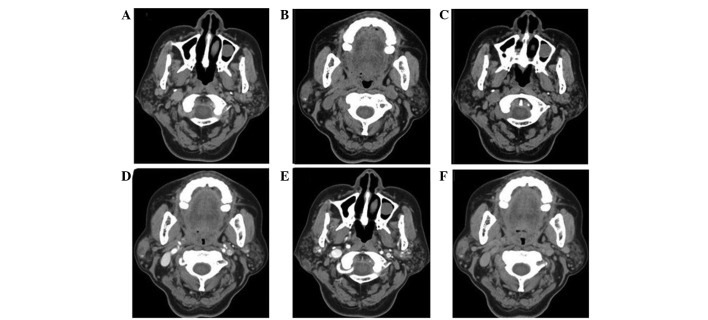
CT of a 68-year-old male patient showing the bilateral parotid gland with increased density and asymmetry. Two oval shaped, unclear foci with necrosis and calcification spots were observed on the right parotid gland. The necrosis was more apparent following CT enhancement. (A and B) Plain CT scan. (C and D) Arterial and (E and F) venous phases. CT, computed tomography.
